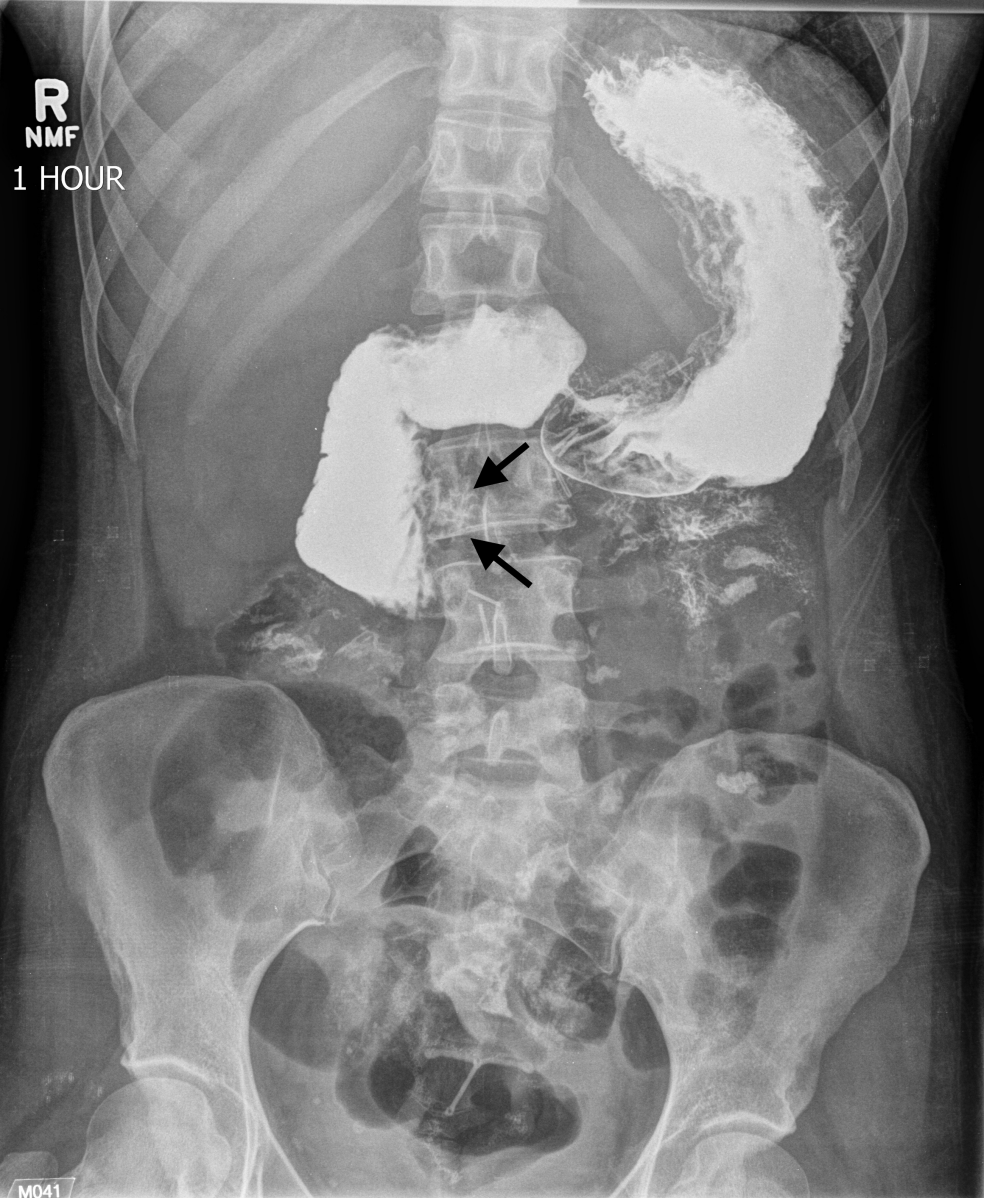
Abdominal radiograph after one-hour demonstrate persistent contrast pooling in the proximal duodenum with an abrupt cut-off at its third part (arrows), coinciding with the superior mesenteric artery impression.
